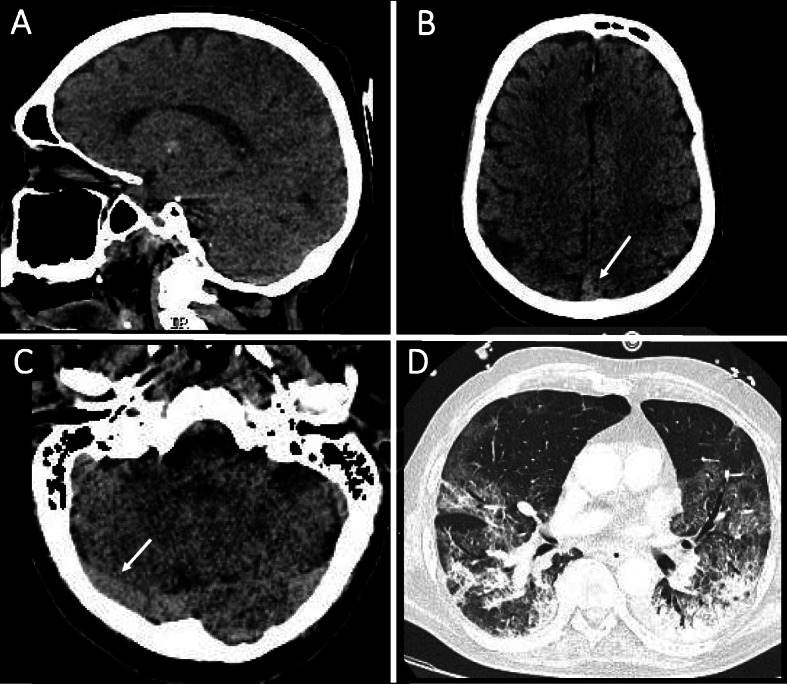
Subacute onset of cerebral sinus venous thrombosis in a man with COVID-19 and ARDS. A Sagittal head NCCT scan showed hyperdense at superior sagittal sinus and right transverse sinuses. B Axial head NCCT scan showed hyperdense at superior sagittal sinus (white arrow) and brain parenchymal edema on the left parietal lobe. C Axial head NCCT scan showed hyperdense at right transverse sinus (white arrow). D Axial thorax CT-scan showed ground-glass opacity and subpleural bilateral, with the fibrotic appearance in both lungs
